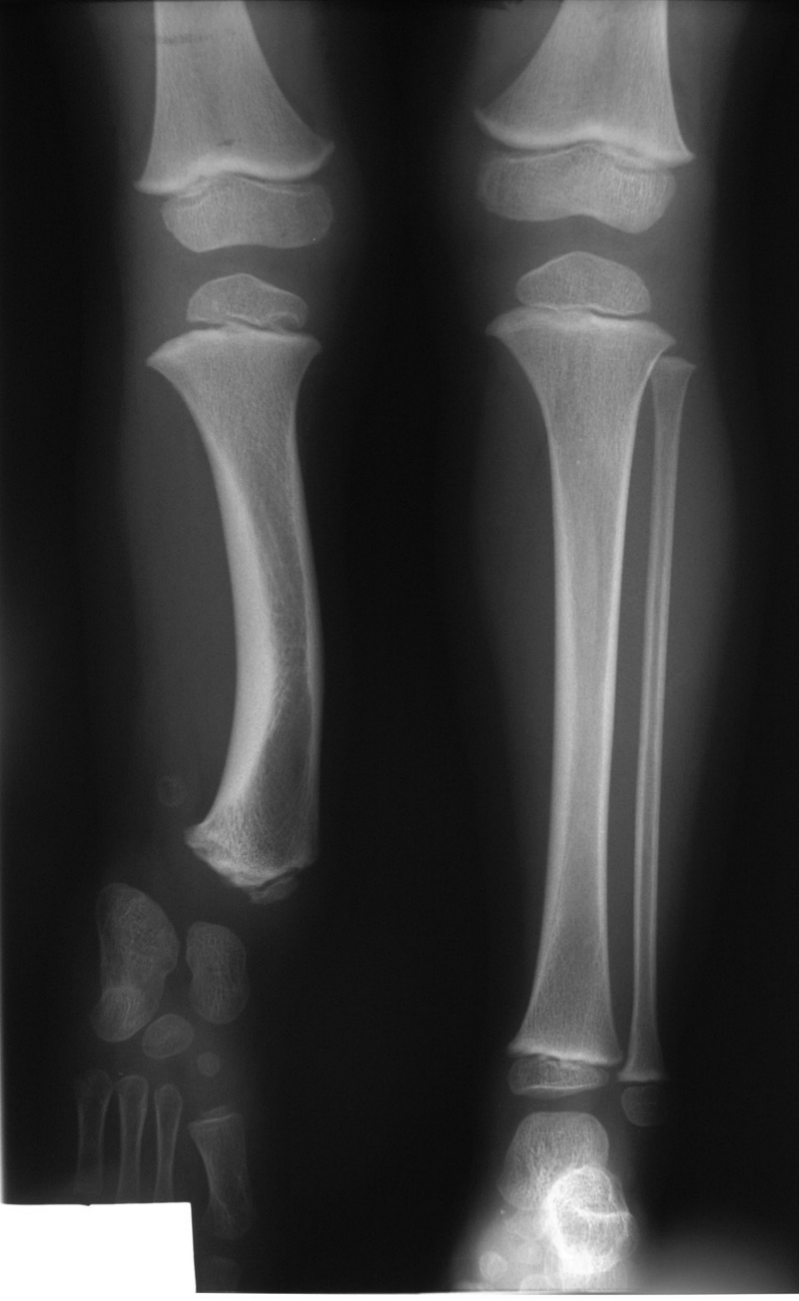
Radiographic presentation of a 6 years old patient with fibular hemimelia type II. Patient's (case 6) radiographs on first examination in the age of 6 years old showing a complete absence of the fibula, antero-medial bowing of the tibia which is significantly shorter compared to the contralateral one and dysplasia of the lateral distal epiphysis of the tibia.
